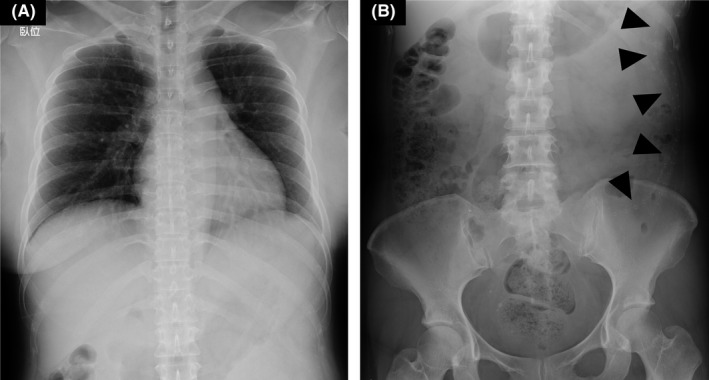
The chest radiograph showing no catheter that should descend subcutaneously through the chest (A), and he abdominal radiograph showing a fractured and migrated catheter in the left peritoneal cavity (B, black arrowheads)
